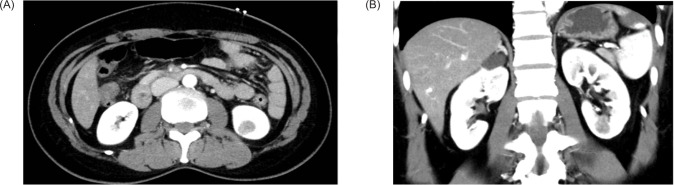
Contrast-enhanced computed tomography images. (A) axial image, (B) coronal image.
